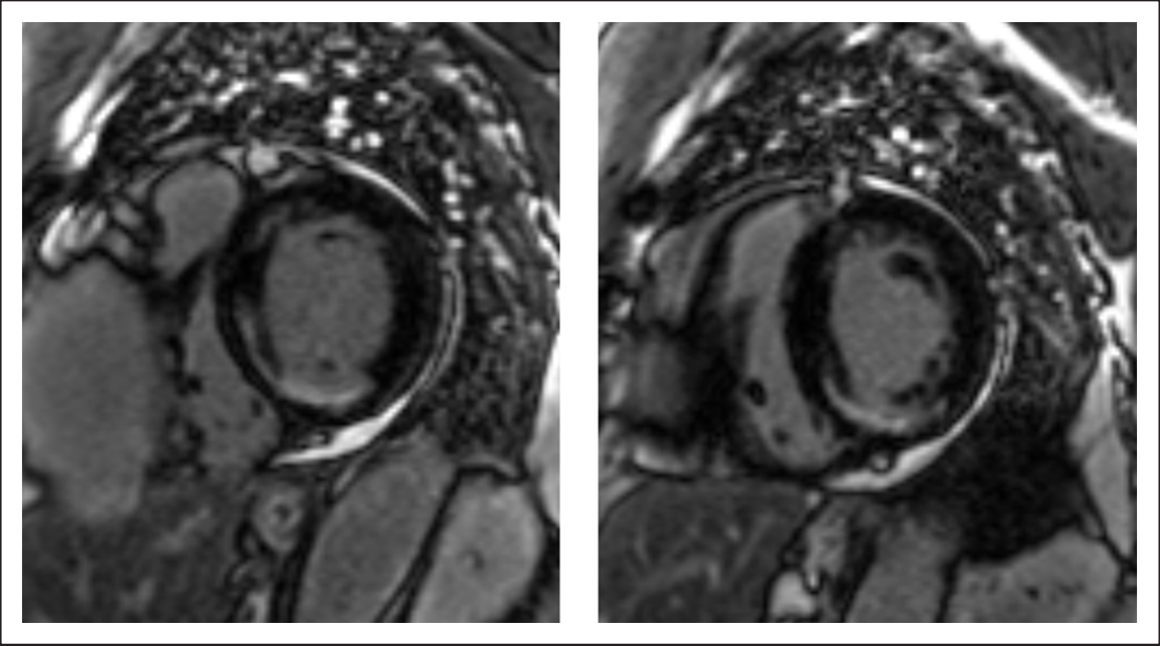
Late gadolinium enhancement cardiac magnetic resonance imaging showing left ventricular subendocardial scar in the basal and mid inferior and inferoseptal walls consistent with right coronary artery related myocardial infarction.
